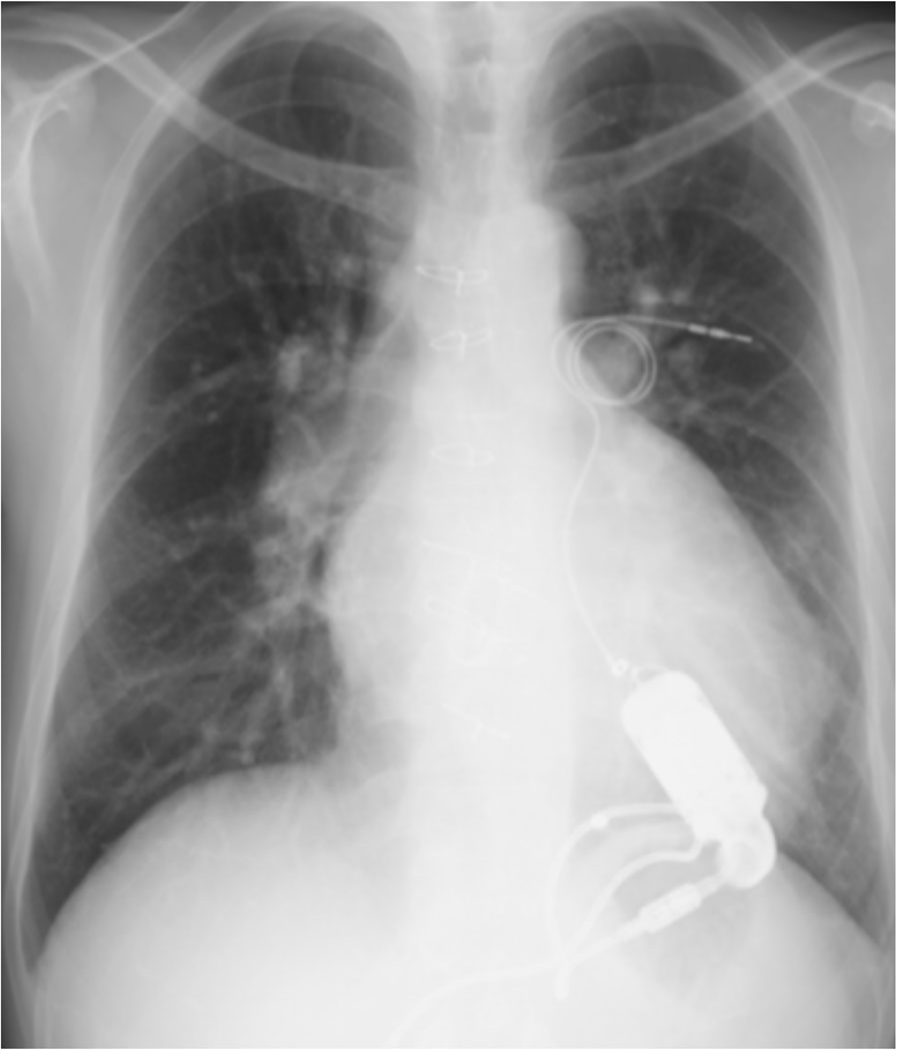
Chest radiography on postoperative day 90 shows a reduced ventricular shadow and the ventricular assist device (VAD)
